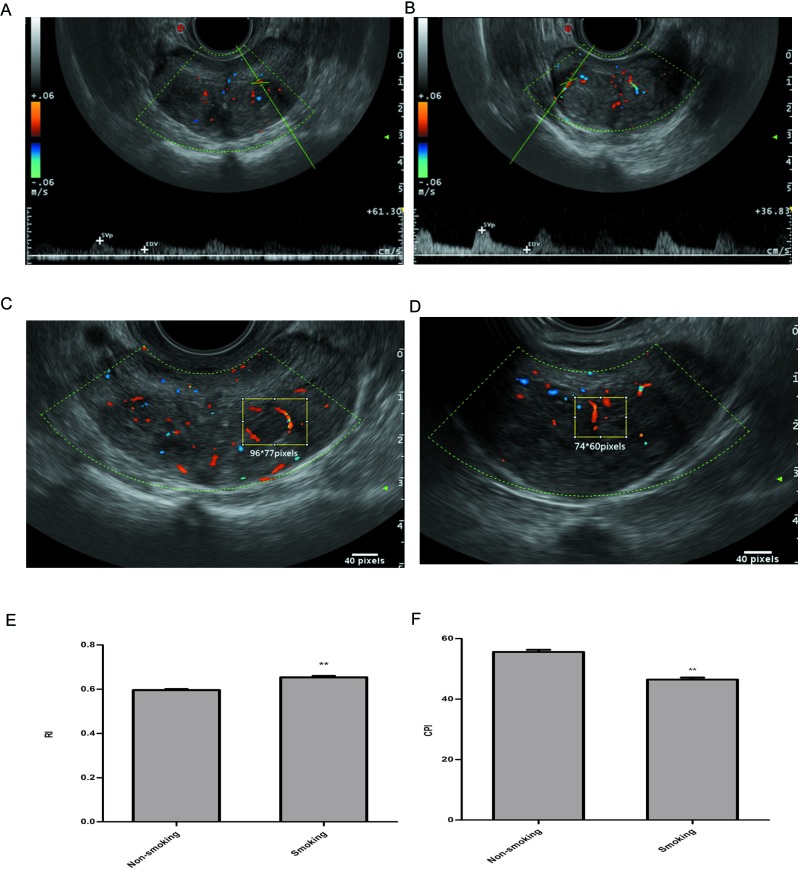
Representative images and analysis of RI and CPI in transrectal colour Doppler ultrasound on prostatic blood flow A. RI for TZ vascular bundles of non-smokers, B. RI for TZ vascular bundles of smokers, C. CPI measurements of non-smokers, D. CPI measurements of smokers E. TZ vascular RI comparison between smokers and non-smokers; F. vascular CPI comparison between smokers and non-smokers; *P < 0.05, *P* < 0.01.
